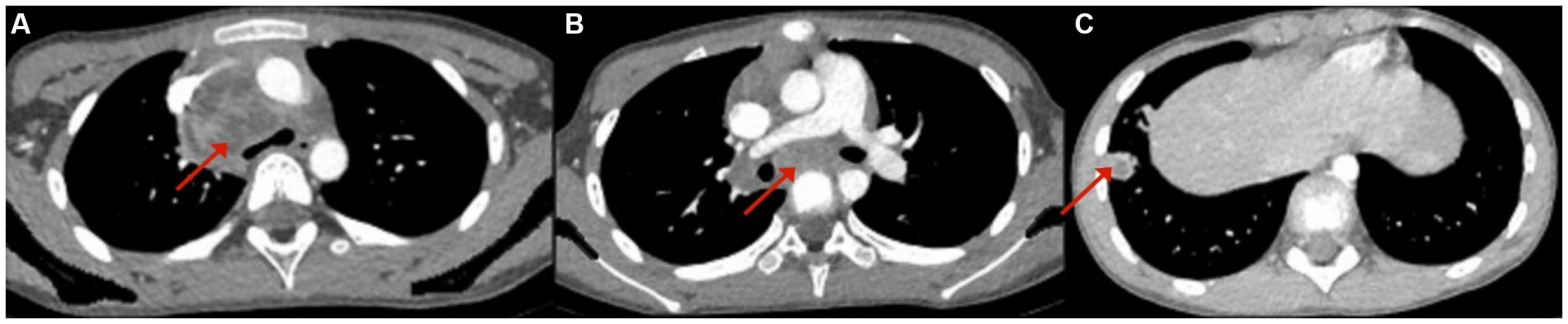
Chest CT: a patchy soft tissue density shadow with obvious uneven enhancement can be seen in the right anterior upper mediastinum, paratracheal vena cava, tracheal prominence, and right pulmonary hilum area, with patchy areas without enhancement visible inside (A,B). The nodular high-density shadow in the anterior basal segment of the lower lobe of the right lung shows annular enhancement (C).
